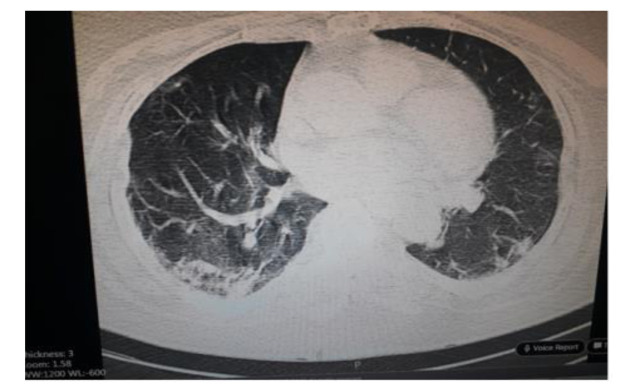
Chest computed tomography scan of a patient with COVID-19
